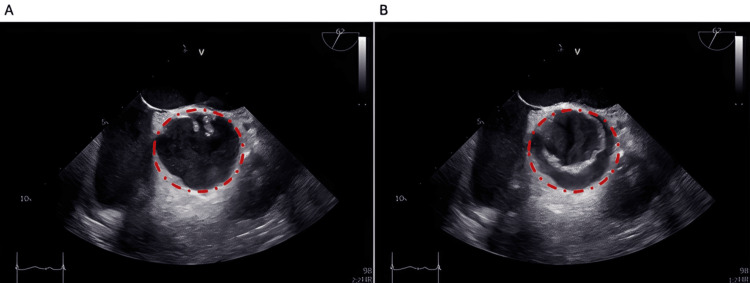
Transesophageal echocardiographic images of a UAV in (A) diastole and (B) systole, obtained in the short-axis aortic view The red dashed circle indicates the aortic valve. UAV, unicuspid aortic valve
